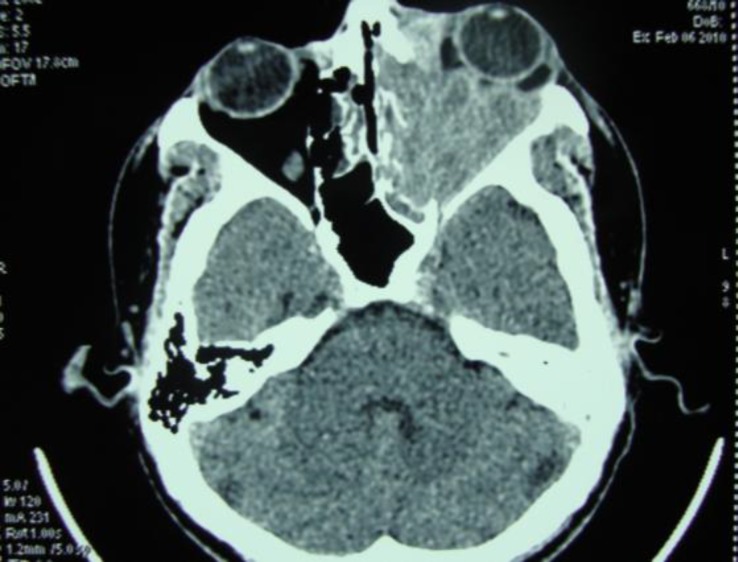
CT Scan PNS showing maxillary mass and proptosis (Case 1
